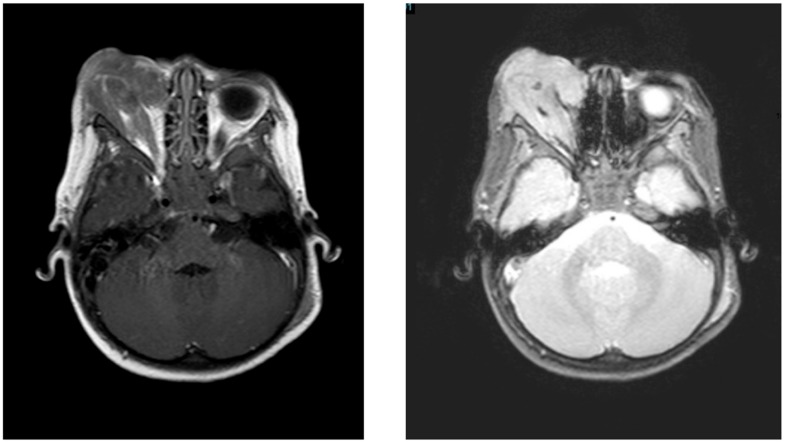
Brain magnetic resonance imaging that shows a prominent mass involving the right orbit. T1 and T2 sequence, respectively.
